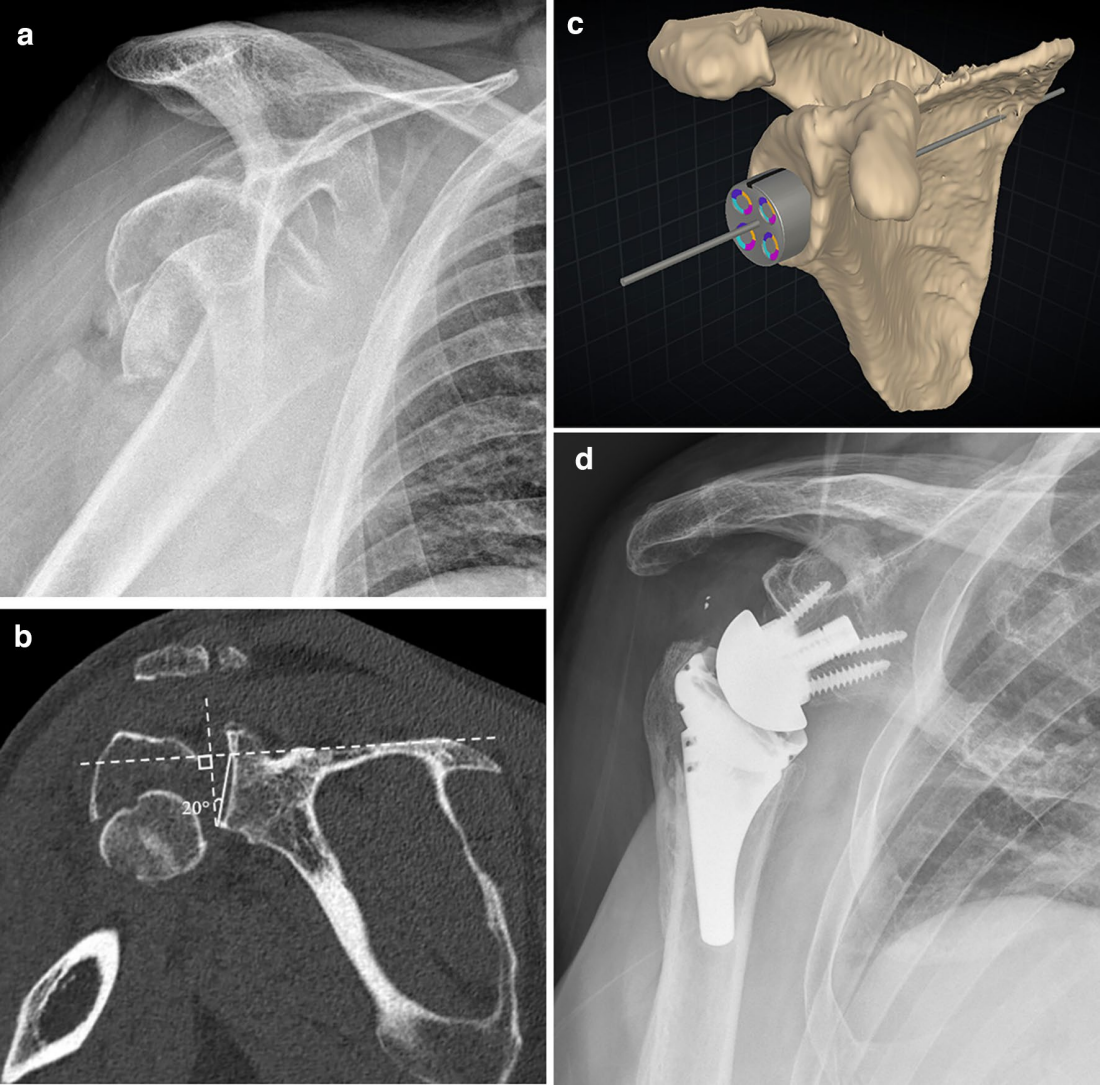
A Scapular Y view radiograph of the shoulder showing a comminuted proximal humeral surgical neck fracture with impaction, displacement, and extension through the greater tuberosity. B Coronal CT image through the scapula and glenohumeral joint in the same patient, again showing the comminuted, displaced proximal humeral fracture, as well as measurement of the RSA angle (20 degrees in this patient). C Reconstructed 3D image of the scapula from prior CT scan on the same patient with preoperative templating software used to determine glenoid baseplate position and size. Due to the patient’s anatomy and specifically the RSA angle, an augmented component was utilized. D Grashey radiograph of the right shoulder in the same patient status post rTSA placement demonstrates appropriate position and alignment of the prosthesis, specifically with neutral to slightly inferior alignment of the baseplate. Note is made of incidental metal debris in the subacromial area
